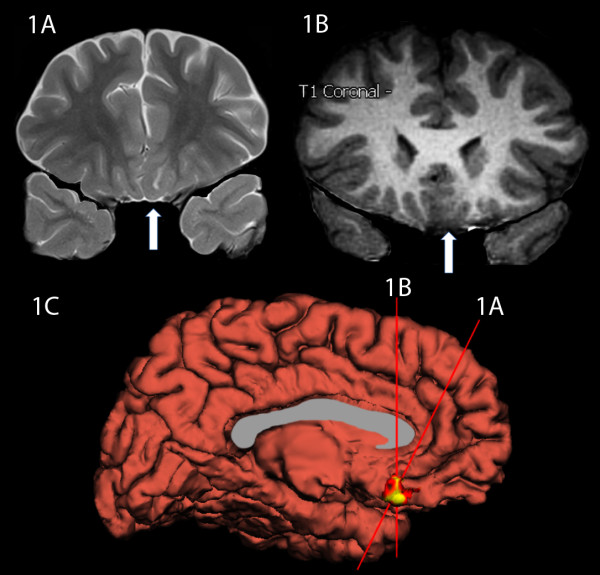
MRI Images. 1A. This is an oblique coronal T2 image at the level immediately anterior to the horn of the lateral ventricles. Note the hyperintense white matter just deep to the gyrus rectus (indicated by arrow) with a linear extension tapering as it courses toward the anterior horn of the ventricle. Also note the cortical thickening of the left gyrus rectus relative to the right gyrus rectus. 1B. This coronal T1 MPRAGE image shows thickening of the left ventromedial prefrontal cortex and blurring of the gray-white interface in this same region. 1C. This is a surface rendering of B.W.'s brain viewing the medial left hemisphere surface with thickened cortex highlighted, which approximates the lesion site. See the appendix for further information on 1C.
